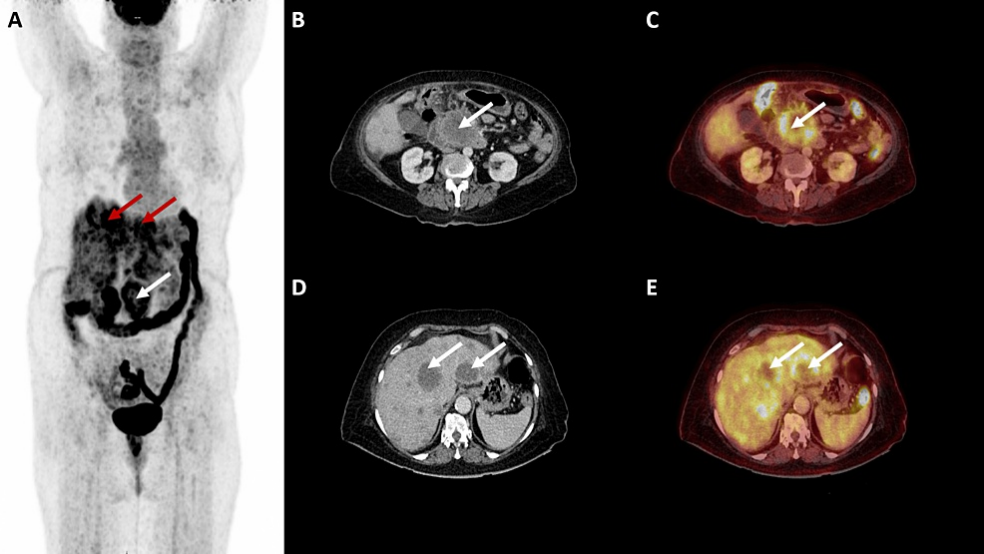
18F-FDG PET/CT images of the patient (A) MIP; (B, D) axial view of CT; (C, E) axial view of 18F-FDG PET/CT fusion. MIP image shows increased FDG uptake in the region of the pancreas (white arrows) and metastatic liver lesions (red arrows). (B, C) Primary neoplastic lesion involving head and uncinate process of pancreas with FDG uptake (white arrows). (D, E) Metastatic liver lesions with FDG uptake (white arrows). MIP: maximal intensity projection; 18F-FDG PET/CT: 18F-flurodeoxyglucose positron emission tomography/computed tomography; FDG: flurodeoxyglucose.
